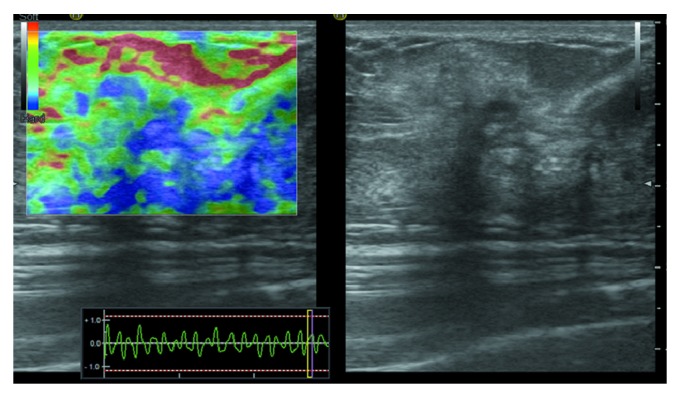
Ultrasound elastographic imaging showed very low strain value in the tumor and the surrounding area, scored as ES 5 based on the 5-point scoring system.
